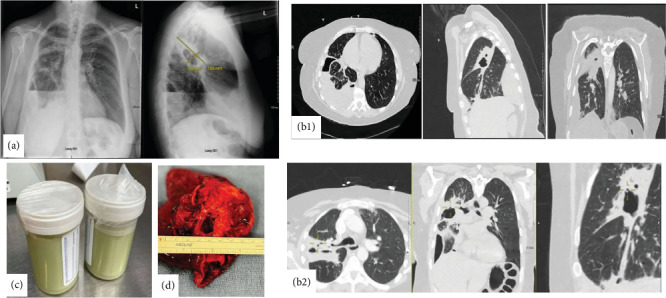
(a) Initial chest X-ray upon presentation showing extensive heterogeneous right lung opacity, which appears to be a thick-walled cavitary lesion about 4.8 × 10.5 cm. Moderate right loculated hydropneumothorax at the lung base with associated air-fluid level, concerning for empyema. (b) b1: A thick-walled cavitary consolidation or lesion in the posterior right upper lobe. There is a complex moderate right pleural effusion with air-fluid levels, concerning for empyema. There is extensive parabronchial thickening in the right lung. b2: CT chest after chest tube insertion; the yellow marker is pointing to a bronchopulmonary fistula, which was the reason for her continuous air leakage. (c) Frank pus after inserting the chest tube. (d) Right upper lobe abscess after lobectomy.
